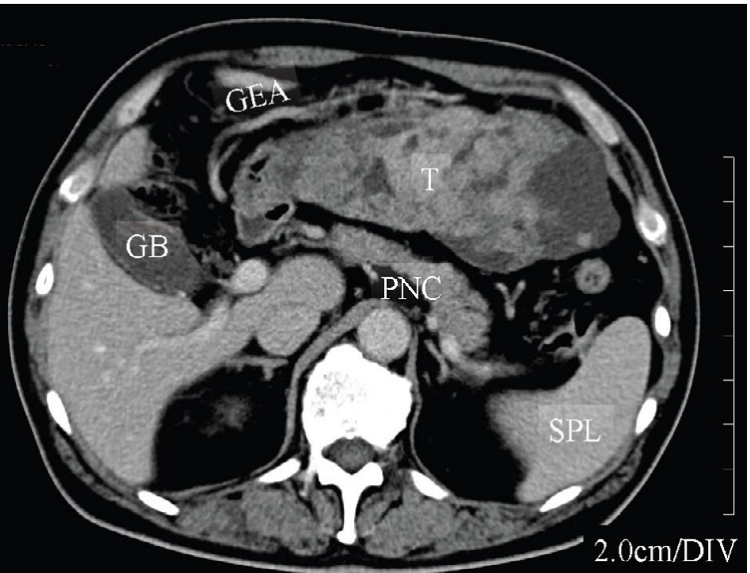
Computed Tomography. A huge tumor behind the left hepatic lobe showed heterogeneous low density with faint enhancement. GB, gallbladder; GEA, gastroepiploic artery; T, tumor; PNC, pancreas; SPL, spleen.
